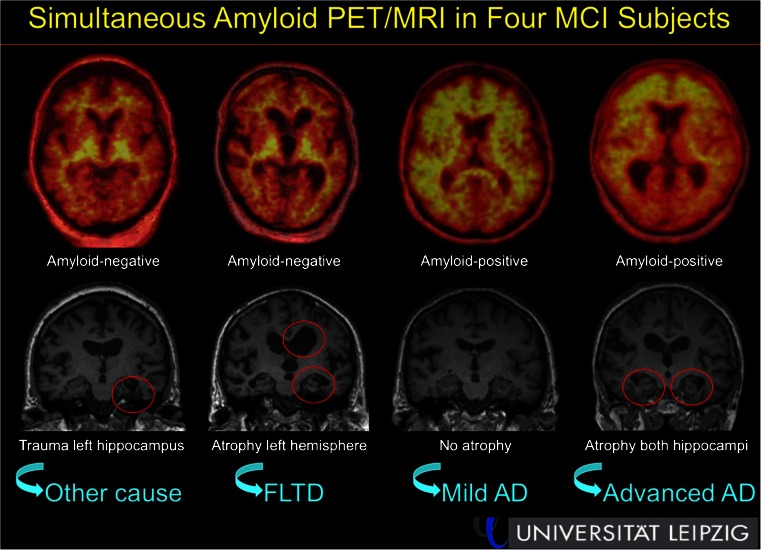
Simultaneous T1-weighted and ^18^F-florbetaben amyloid PET/MRI in MCI patients, allowing improved differential diagnosis and prognosis assessment in a one-stop-shop fashion [36]
